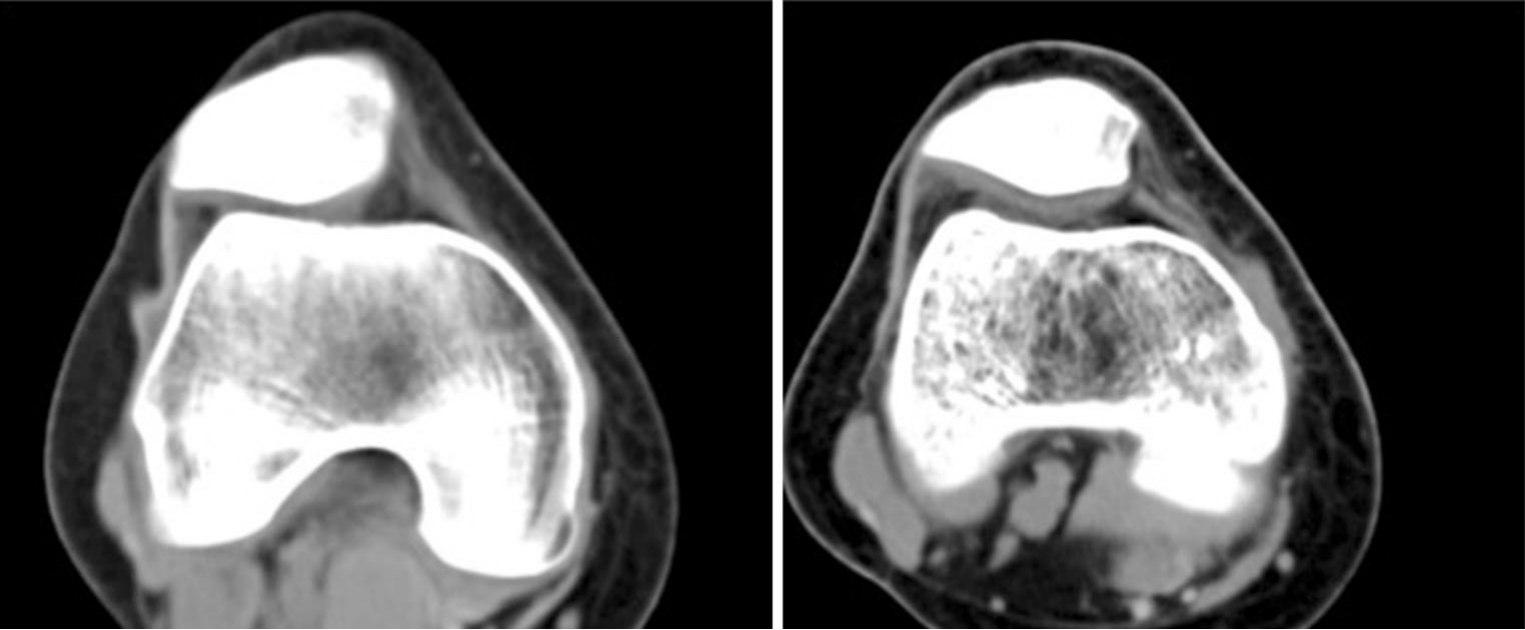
CT scan showing the correction of patellar maltracking after a surgical procedure
